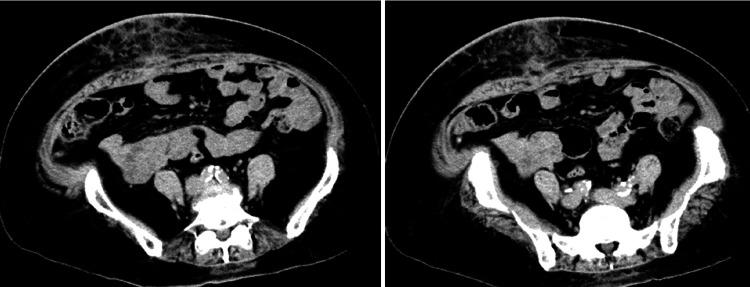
Postoperative CT scan findings A 45-day postoperative CT scan showed no abdominal wall dehiscence. CT: computed tomography
